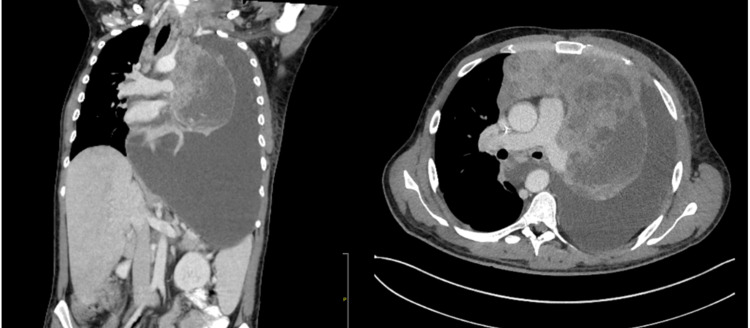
Axial and sagittal computed tomography images at the time of presentation of the large anterior mediastinal mass with complete left lung atelectasis and associated large left-sided pleural effusion
